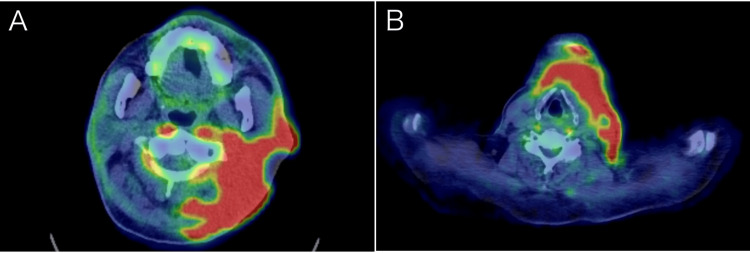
An 18F-fluorodeoxyglucose positron emission tomography (FDG-PET) performed just prior to the third period of admission. (A) Left-sided head and (B) left anterior neck showing strong accumulation of FDG from the skin to deeper tissues.
